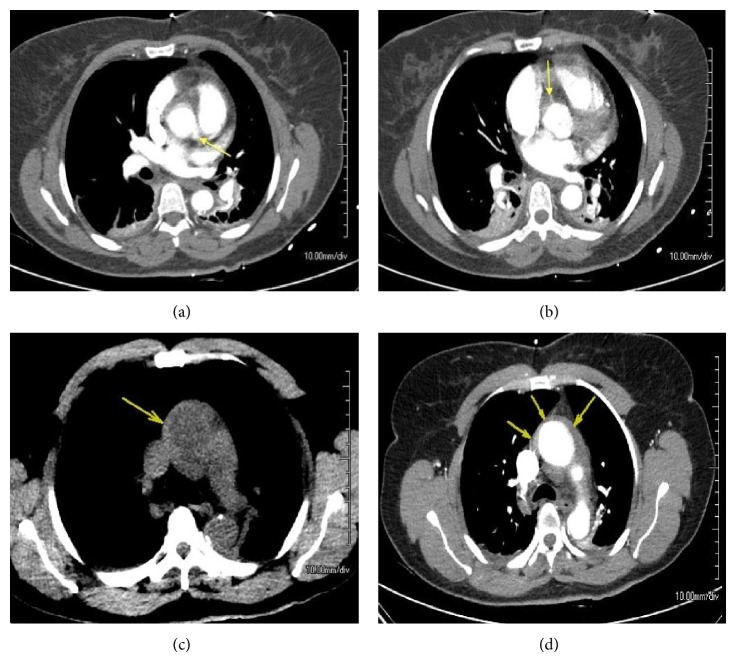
(a)-(b) CT angiogram at the level of the LCA and RCA, respectively (yellow arrows). (c)-(d) Arrows indicate the area of increased intensity suspicious for hemorrhage before (c) and after (d) contrast injection.
